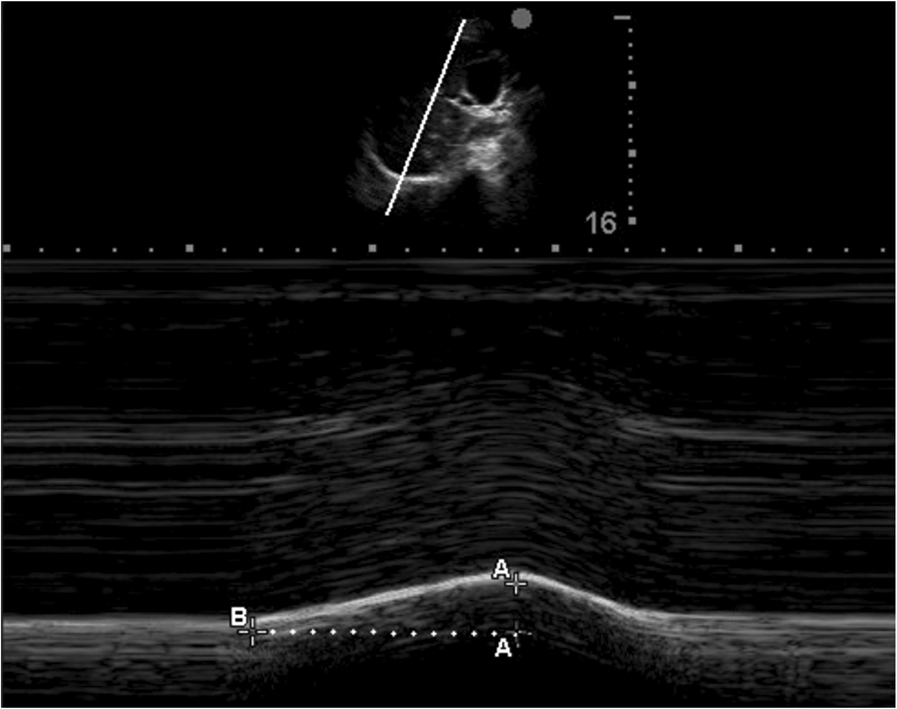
Image showing measurement of the diaphragmatic inspiratory excursion and TPIA_dia_ in M-mode ultrasound. The A-A is the height of diaphragmatic inspiratory excursion. The B-A is the TPIA_dia_. TPIA_dia_, time to peak inspiratory amplitude
